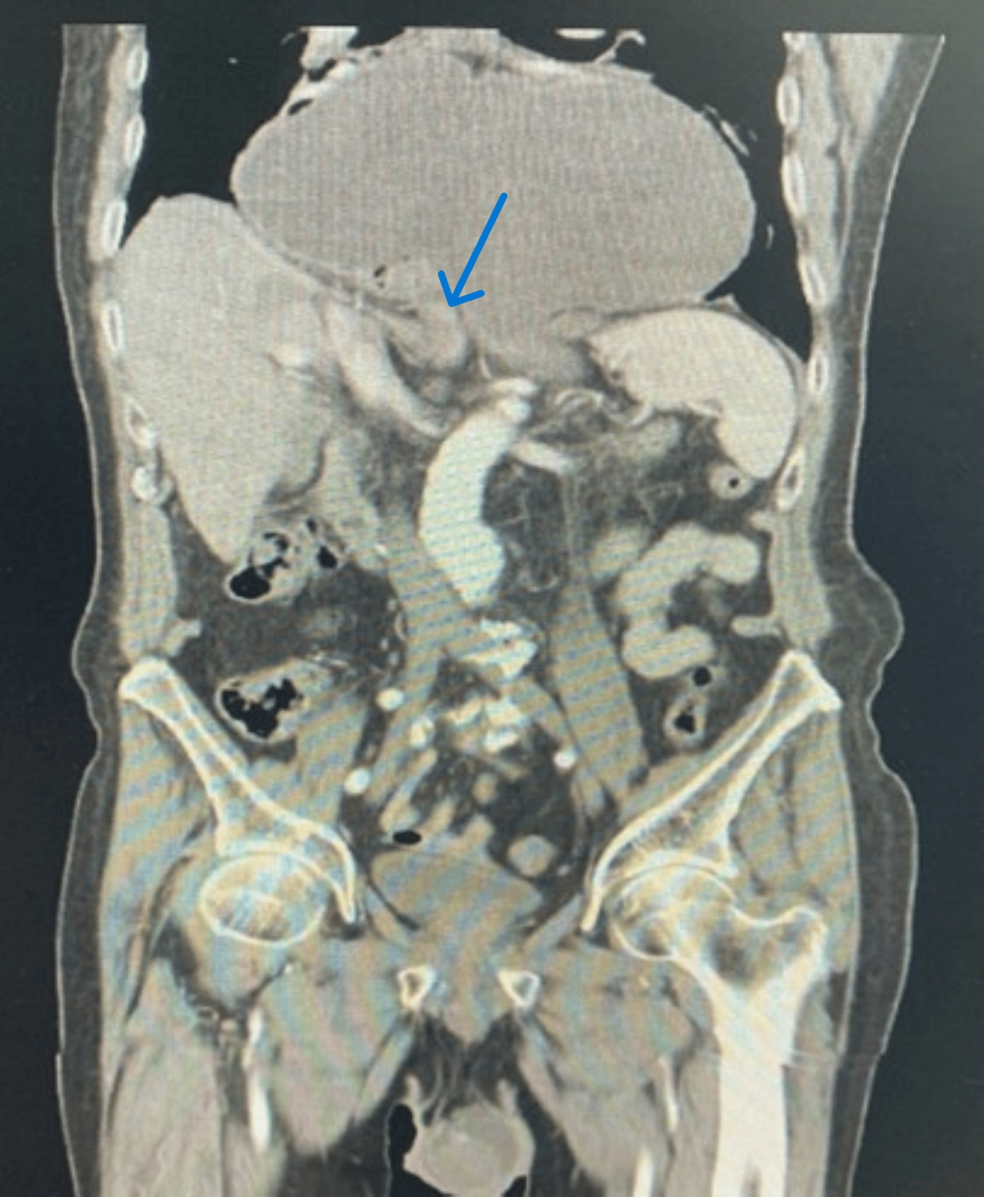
CT scan showing mixed-type incarcerated hiatal hernia with the blue arrow pointing at the pylorus CT: computed tomography
